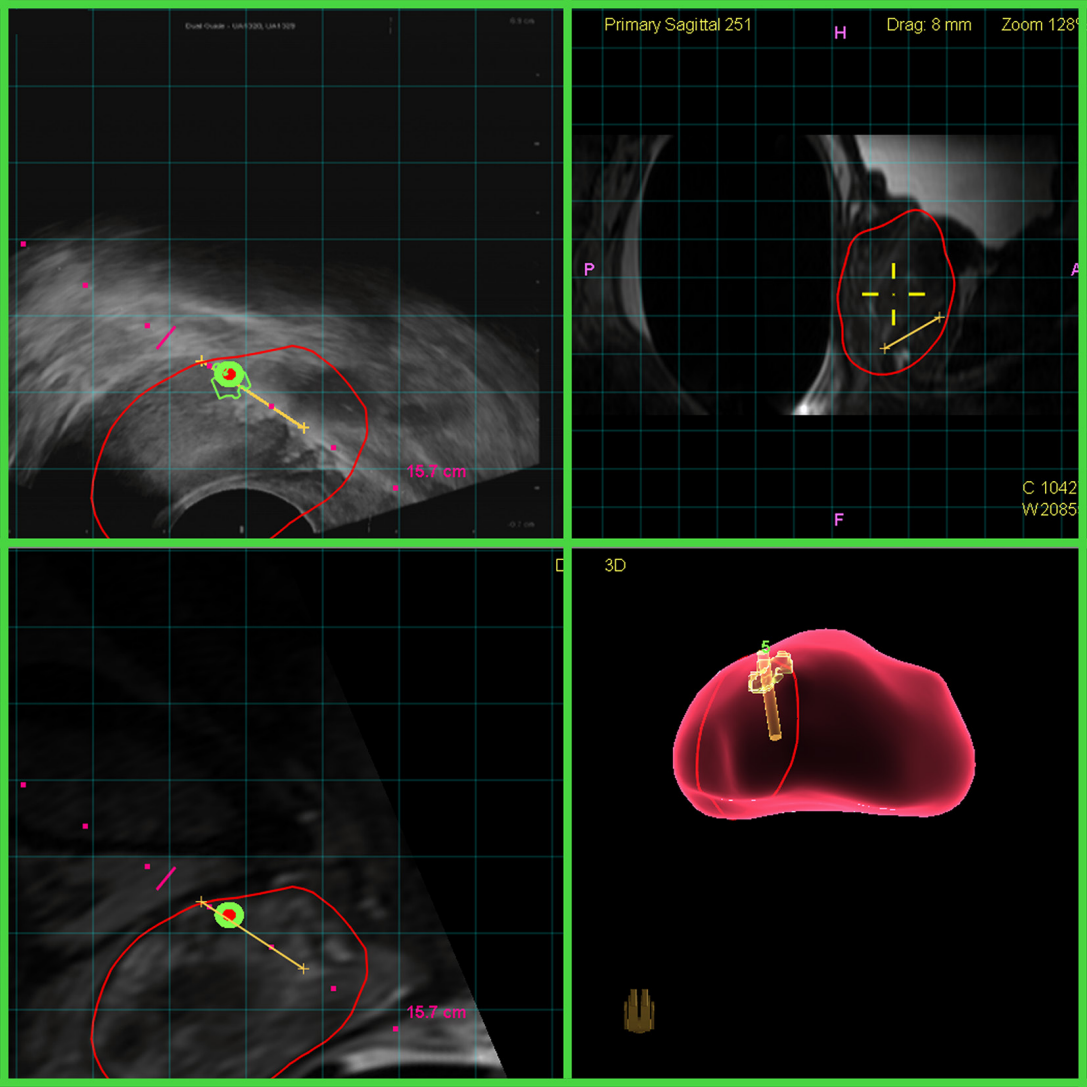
MRI-TRUS fusion targeting. The green and red circles seen in the squares of the left represent the center of the target lesion identified on MRI (bottom left), transposed to the real-time TRUS image (top left). An oblique sagittal reconstruction of the MRI is shown in the top right square. The right bottom square contains the needle tracking map. This allows the successful biopsy of targets such as the one depicted in Figure 3. TRUS,transrectal ultrasound.
